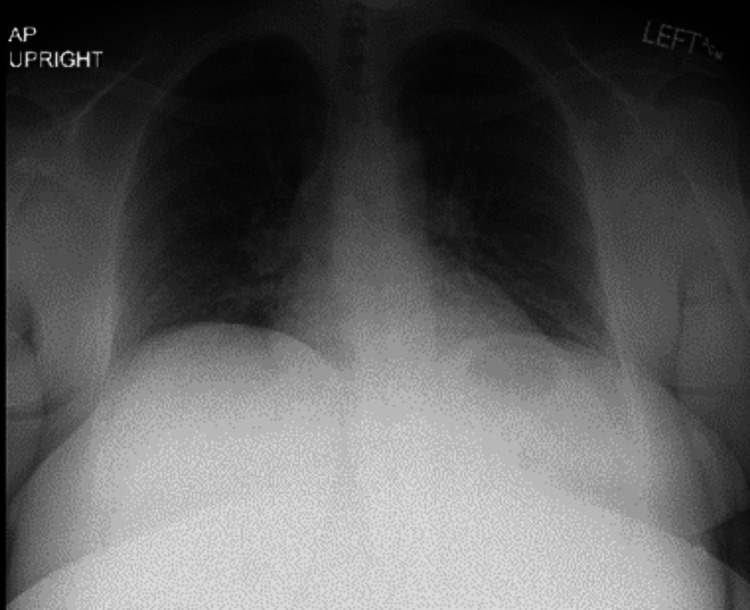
A chest X-ray conducted nearly 1.5 years later, depicting substantial improvement in lung condition
